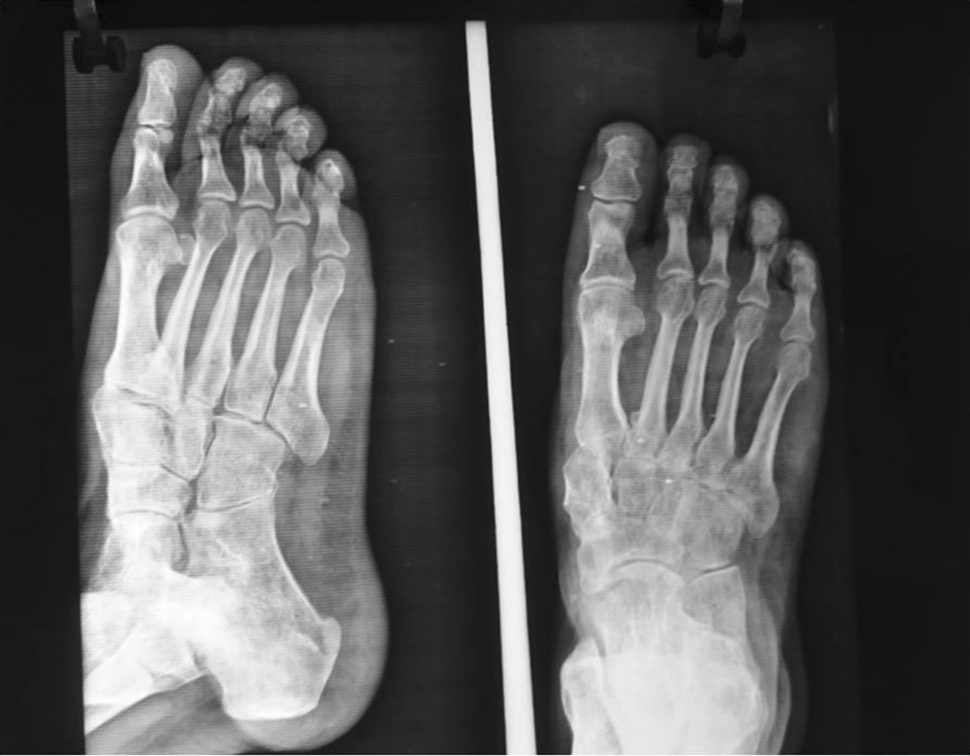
X-rays–AP and oblique view of the foot at 3-month follow-up
